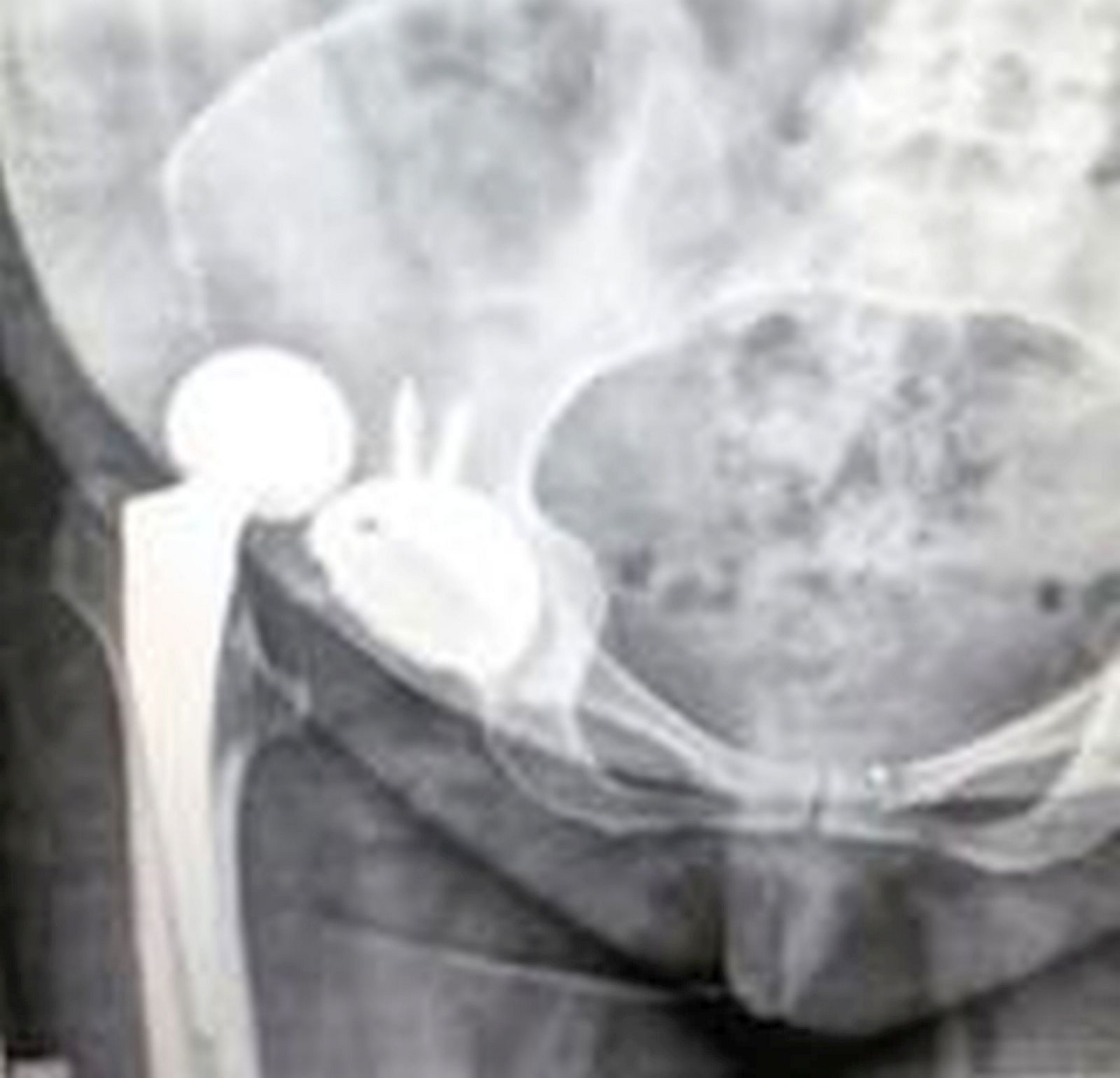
One month postoperative X-Ray of right hip. Anteroposterior view (AP) showing dislocated total hip replacement.
